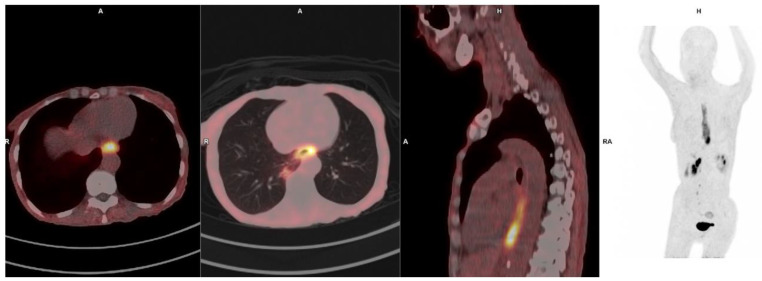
65-year-old female with SCC of the distal esophagus; ^68^Ga-FAPI-46 PET/CT demonstrates intense tracer accumulation in an extensive lesion extending from T2–T10 with no distant metastasis.
